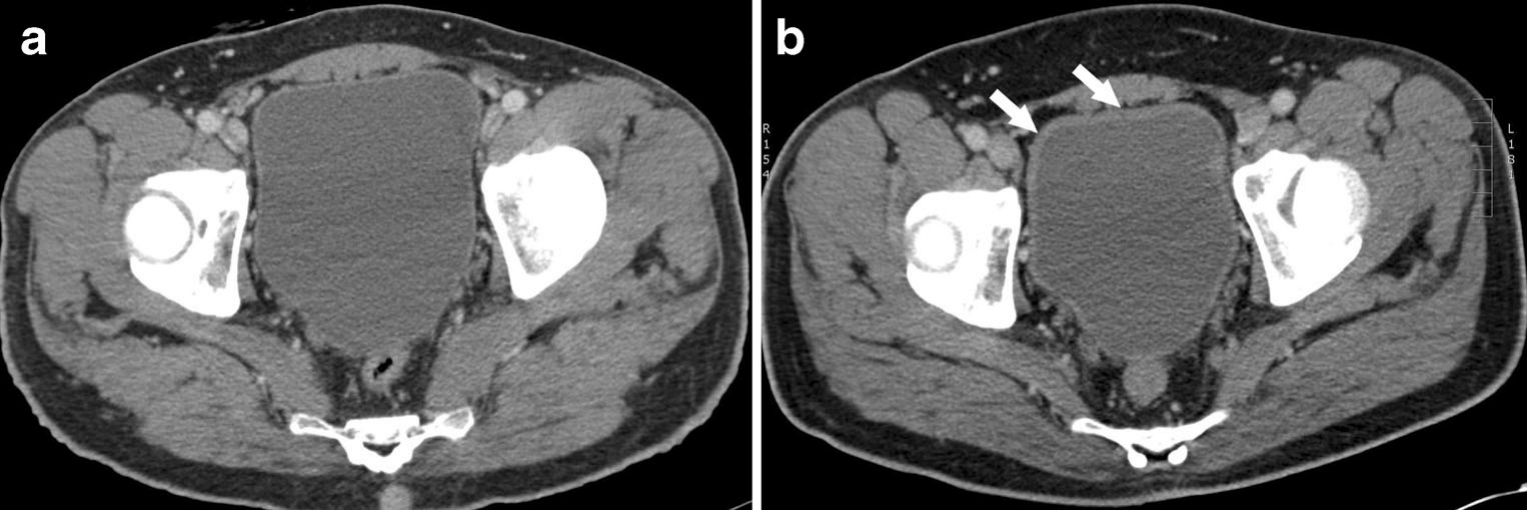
Forty-four-year-old male with non-Hodgkin’s lymphoma status post treatment with cyclophosphamide. (a) Axial CT pre-chemotherapy image showing normal bladder findings. (b) Axial CT image showing thickened urinary bladder wall consistent with chemotherapy induced hemorrhagic cystitis (*arrows*)
